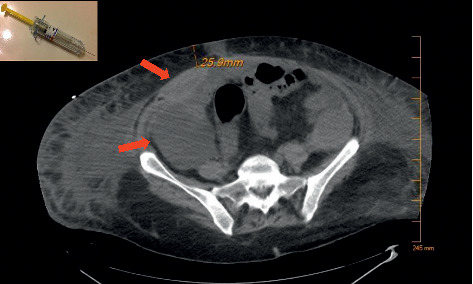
CT performed on day 8 showing right-sided rectus sheath and pelvic hematomas. The inferior epigastric artery was measured approximately 25.9 mm below the skin. A standard enoxaparin injection needle at our facility has a length of 15.9 mm. Photo of the needle was adapted from https://tinylittlehuman.wordpress.com/2012/11/02/lovenox/.
